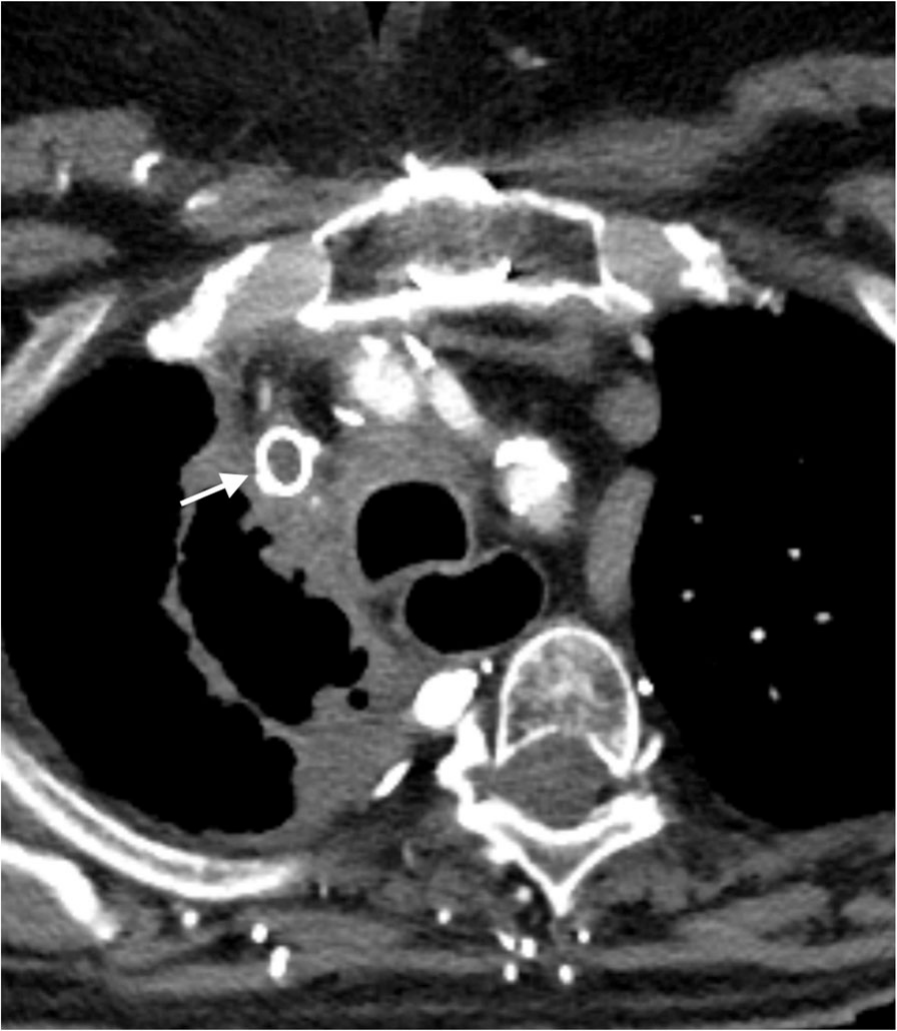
Thrombosed stent. Axial CT image demonstrating a patient who has a thrombosed stent within the SVC (arrow)
